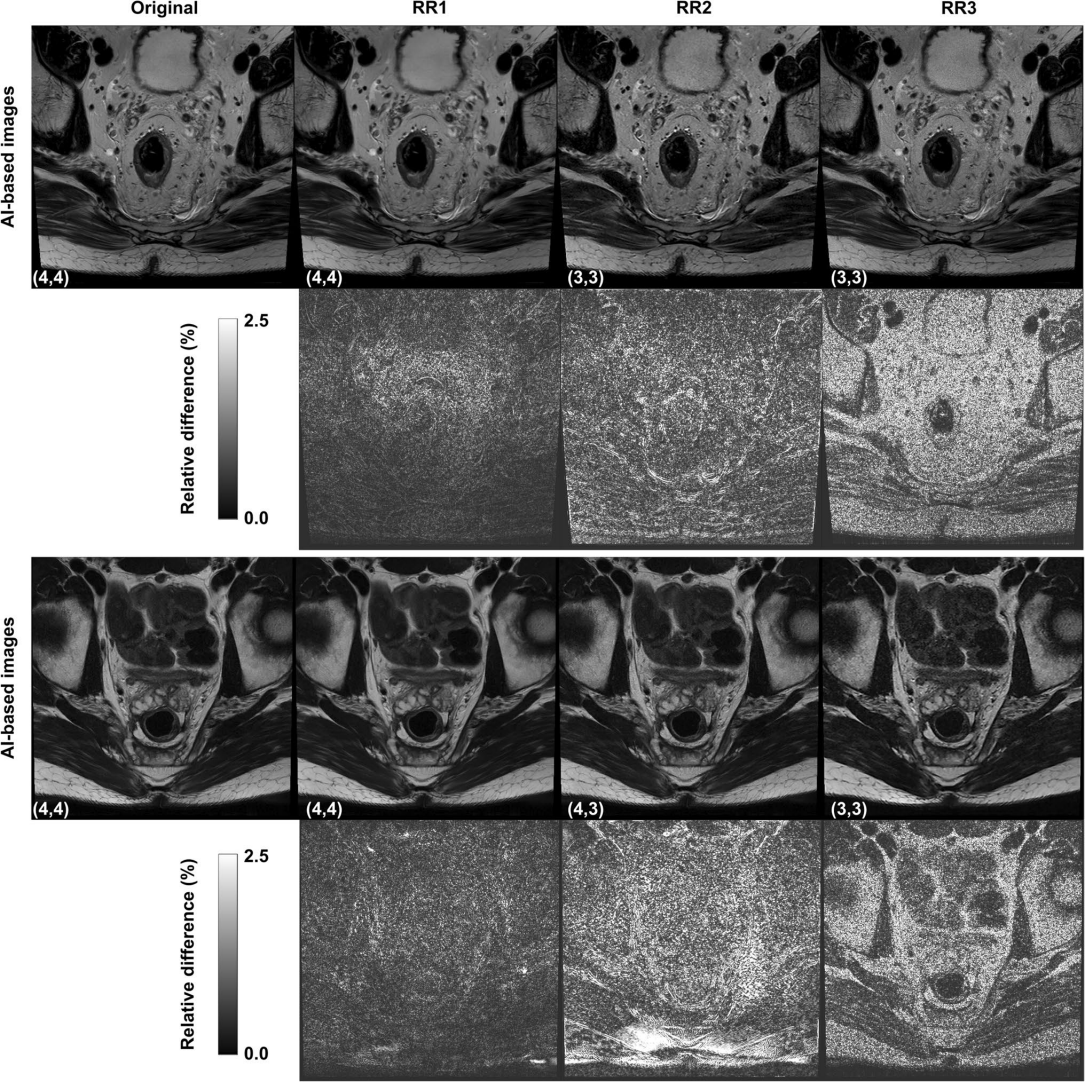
Example axial T2-weighted turbo spin echo (TSE) images acquired using the AI-based reconstruction technique for two patients. Top rows: a 56-year-old male patient treated with chemoradiotherapy for a T2-stage rectal cancer and subsequent complete clinical response, scanned at 3 T. Bottom rows: a 36-year-old male patient treated with chemoradiotherapy for T2-stage rectal cancer and subsequent good partial response, scanned at 1.5 T. Both patients were on a “watch and wait” active surveillance protocol. The original reconstruction and retrospective reconstruction (RR) images are shown. The RR reconstructions are calculated with deliberate perturbations: RR1) an increase in the AI-reconstruction mode denoising strength from “medium” to “high”, RR2) a decrease in the AI-reconstruction mode denoising strength from “medium” to “low”, and RR3) an additional image edge enhancement and smoothing filter. The Radiologist scores for overall image quality are displayed in the corners of the images (Radiologist 1, Radiologist 2). Note that these scores were measured per series, not per slice. Relative difference images calculated between the original images and RR images are shown. The relative difference images are calculated relative to the maximum intensity of the original image and displayed as a percentage, i.e. $$\left|\text{Original image - Perturbed image}\right|/\text{max(Original image)}$$
